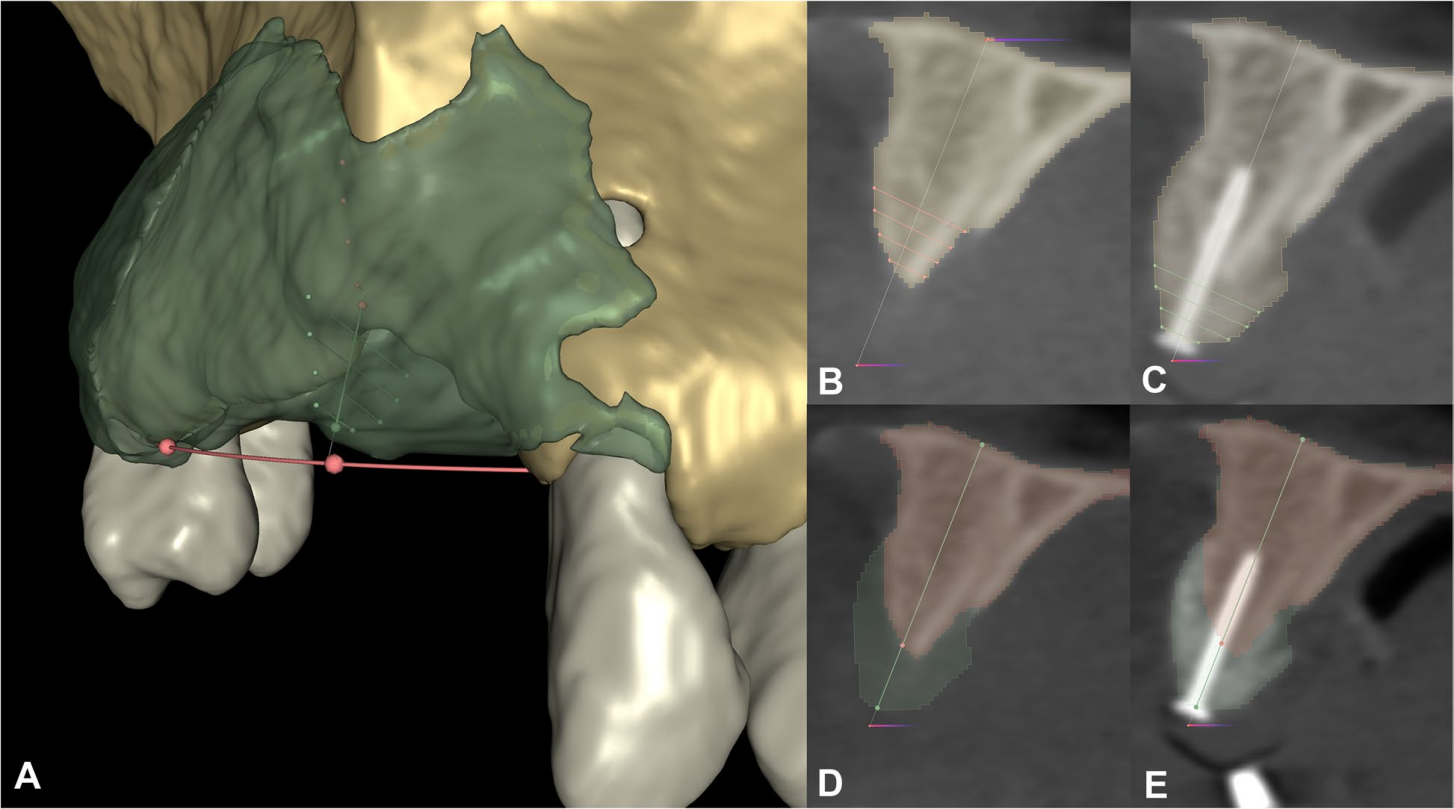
Vertical- and horizontal linear measurements at the greatest of hard tissue gain (Participant 7). A Linear measurements visible on a virtual 3D model. Red open curve placed at the level of the proximal bone of adjacent teeth marks the middle point of the alveolar crest. Preoperative measurements are visible in red, postoperative measurements are visible in green. B-C Baseline and 9-month follow-up horizontal measurements visible on the reoriented planar CBCT image. Horizontal measurements were performed 0,1,2,3 mm-s apical to the marginal crest perpendicular to the white reference line. D-E Baseline and 9-month follow-up horizontal measurements visible on the reoriented planar CBCT image. Vertical measurements were made along the gray reference line. Preoperative alveolar bone marked with a red, postoperative alveolar bone marked with a green labelmap
